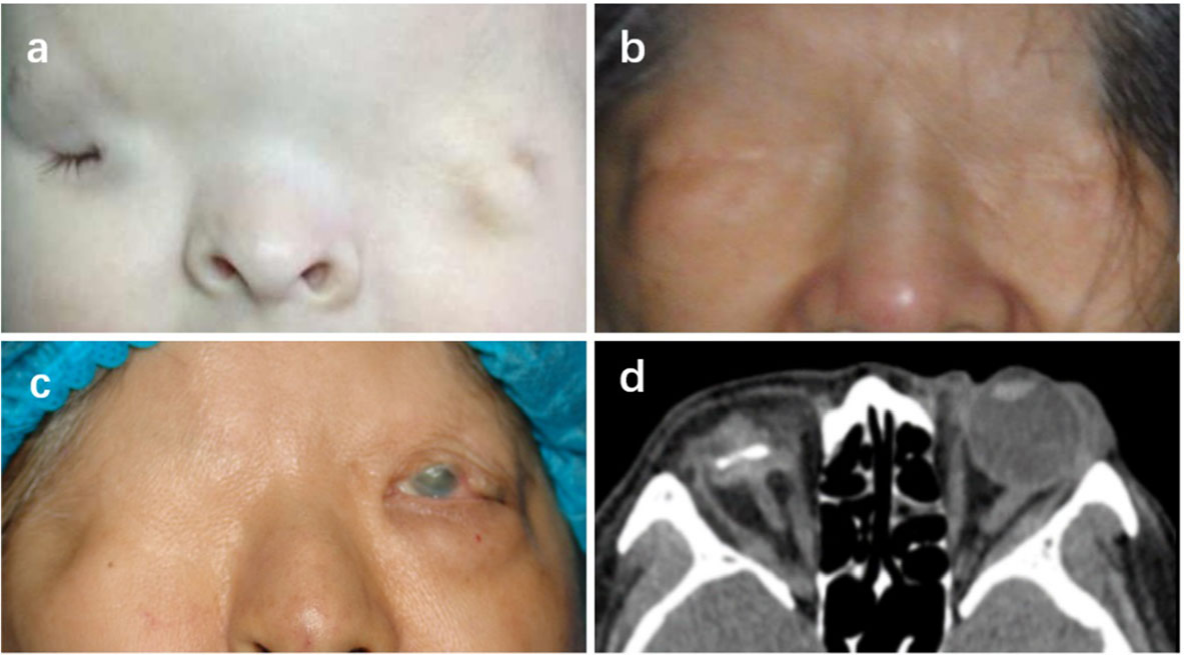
a Case 4, one-month old boy with complete cryptophthalmos OS and incomplete cryptophthalmos OD. b Case 5, the first sibling with bilateral complete cryptophthalmos. c Case 6, the second sibling with complete cryptophthalmos OD and abortive form OS. d CT scan of Case 6 displayed severe disorganized intraocular structure OD
